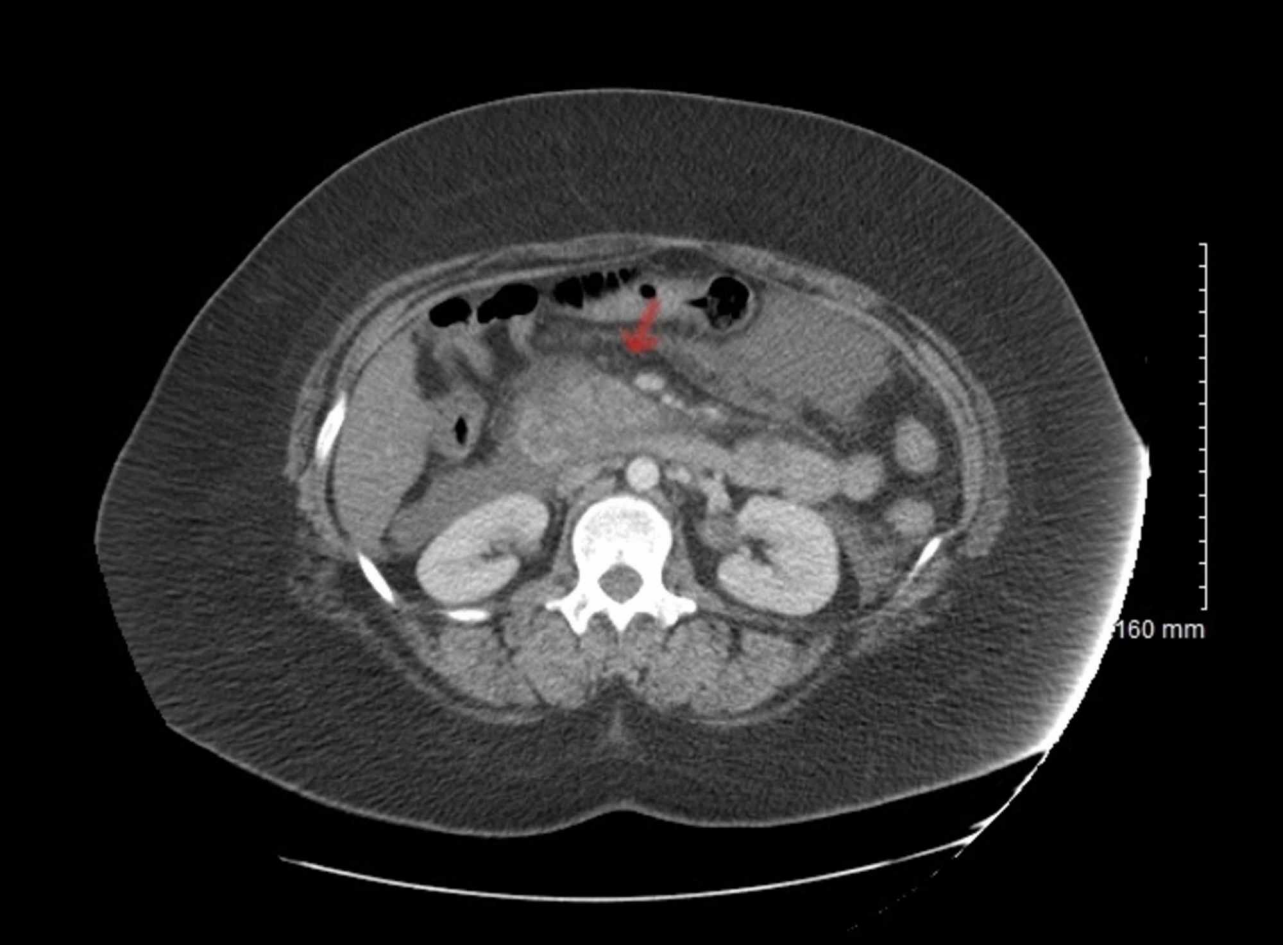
Computed tomography of the abdomen showed diffuse peri pancreatic inflammatory stranding and fluid (red arrow)
